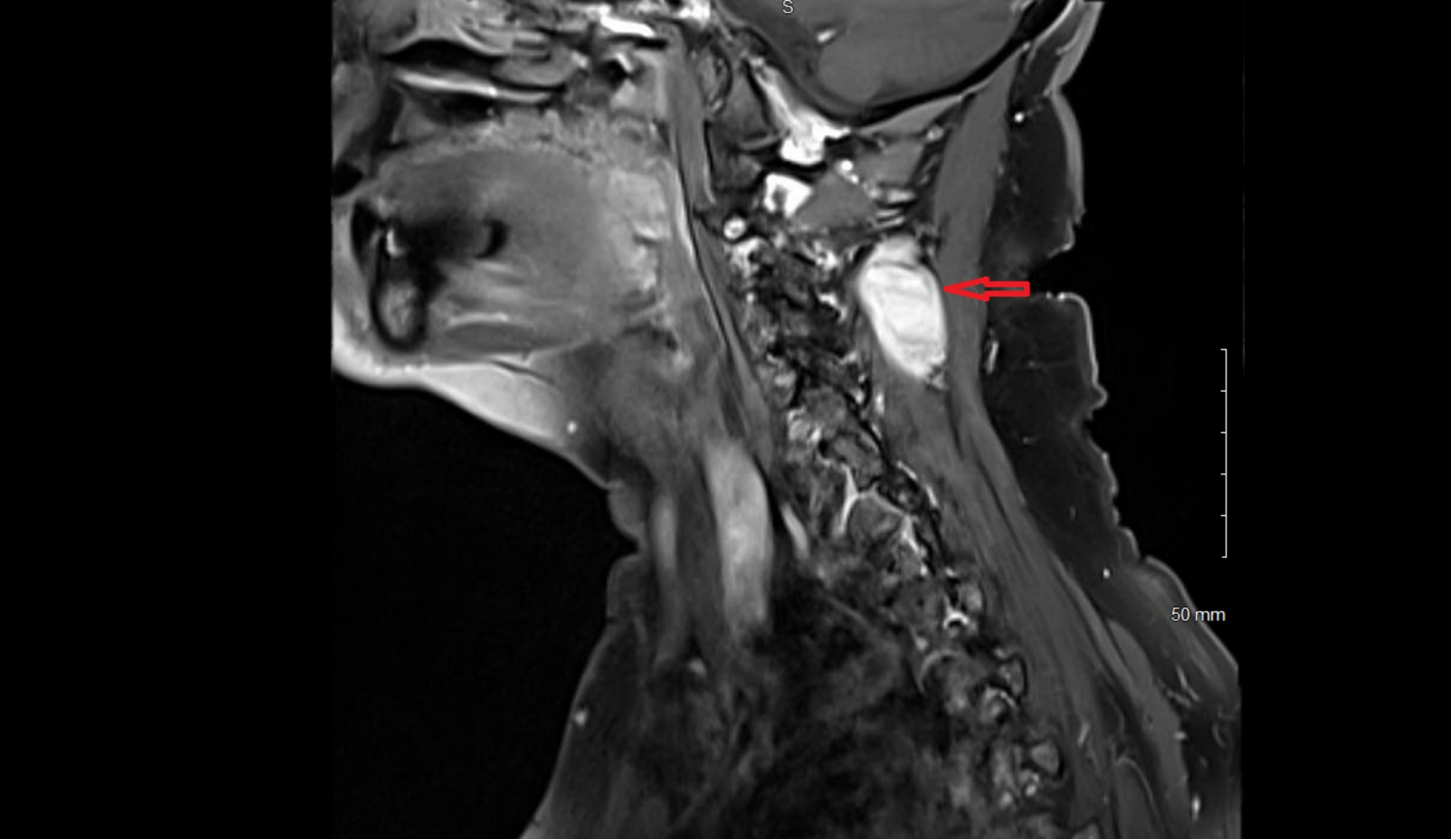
Parasagittal MRI postcontrast. Postcontrast MRI showing enhancement of the mass in the parasagittal plane.
